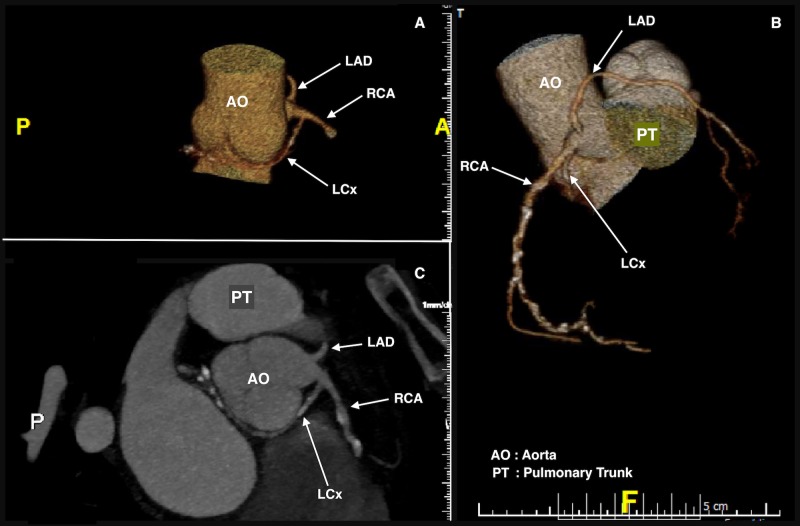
Cardiac Multi-detector Computed Tomography (MDCT) with 3-D reconstruction of the vessels. Panel A- demonstrates the course of the LCx as it swoops around the aorta after originating from a common trunk with the RCA. Panel B- demonstrates the origin and course of the Left Anterior Descending Artery ( LAD) anterior to the RCA  and over the pulmonary trunk. Panel C- computed tomography angiogram of the coronaries demonstrating the origin of the three vessels from the right coronary cusp and their relative locations to each other.
